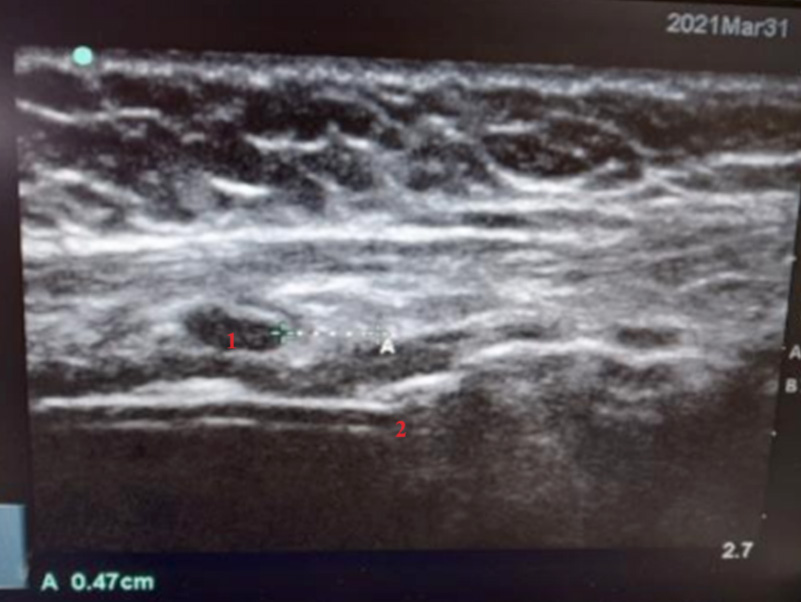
Ultrasound image showing cricoid cartilage (1) and cutoff point (2)
